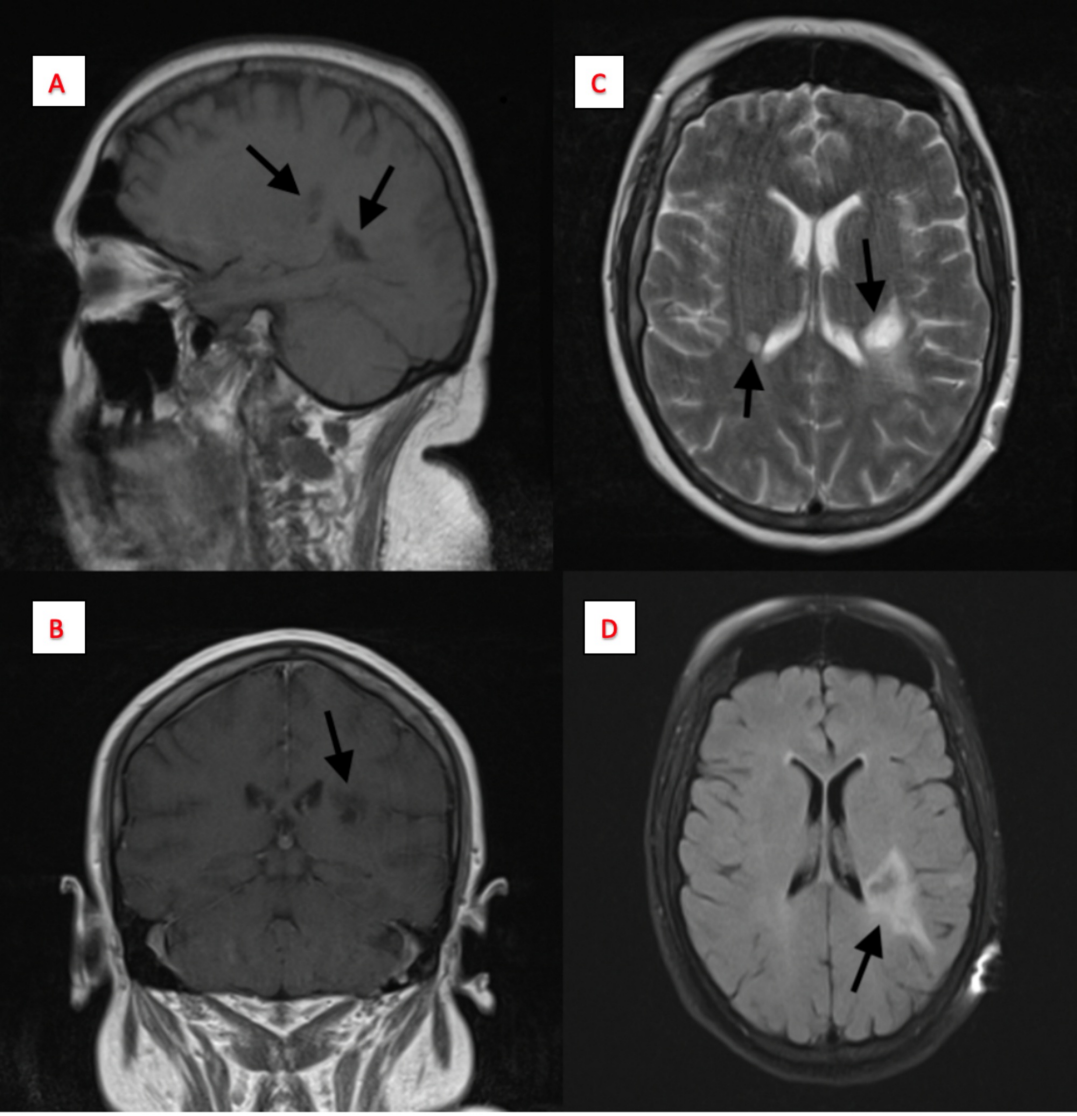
Magnetic resonance imaging (MRI) of the brain with/without contrast four months post-biopsy and treatment Panel A: Displays sagittal T1 image with two reduced hypodense lesions; Panel B: Displays a T1 contrast-enhanced coronal image of reduced lesions in both cerebral hemispheres; Panel C: Displays a T2-weighted image with two reduced periatrial ring-enhancing lesions in the left periatrial white matter; Panel D: Displays the fluid-attenuated inversion recovery (FLAIR) image of reduced periventricular lesion. Note: Tumor dimensions on this scan were not available.
